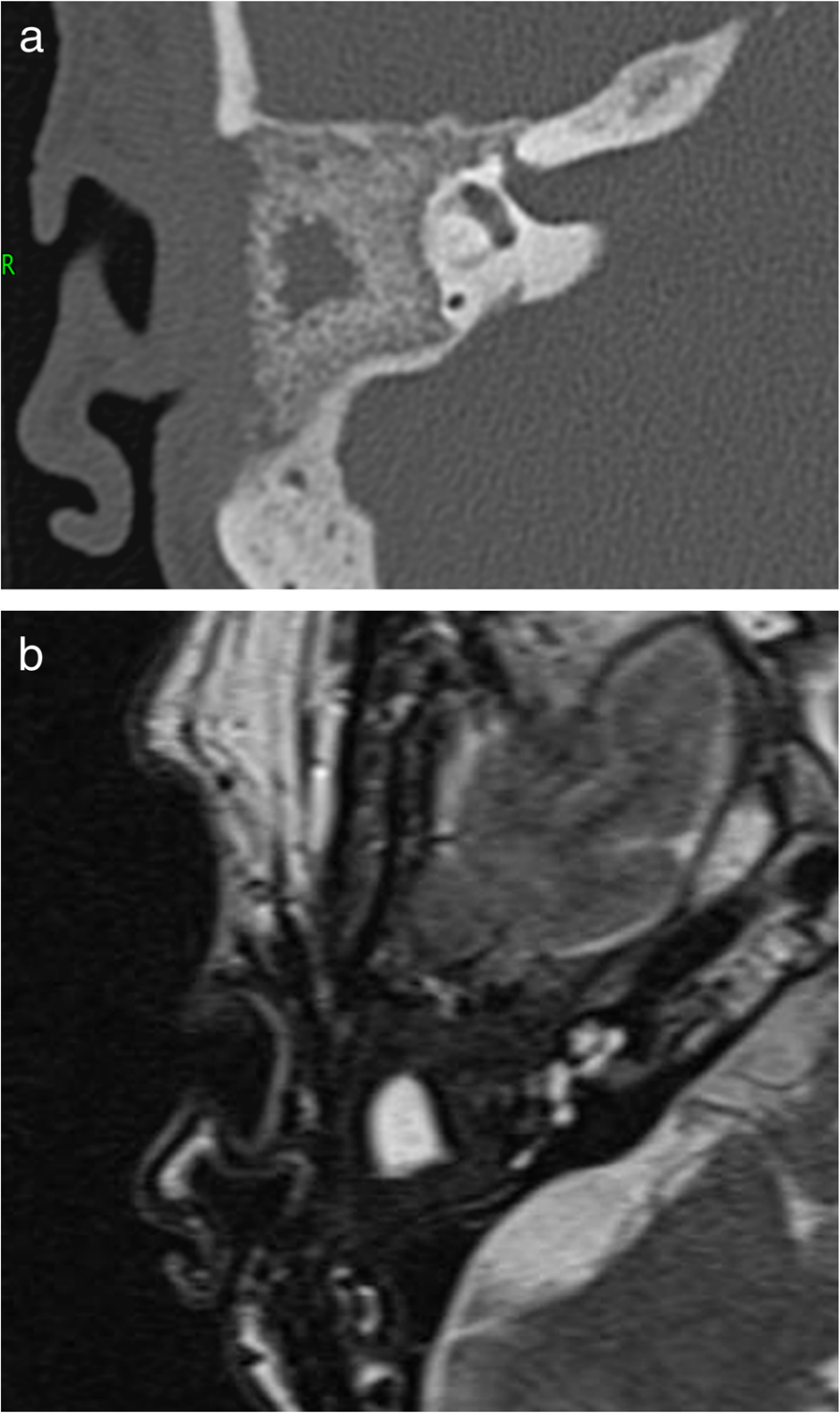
a Axial CT of the right mastoid showing a homogenous distribution of the bioactive glass S53P4 in the cavity of the middle ear and mastoid with a central hypodense area considered to represent seroma or fluid. b T2-weighted axial MRI of the same level
